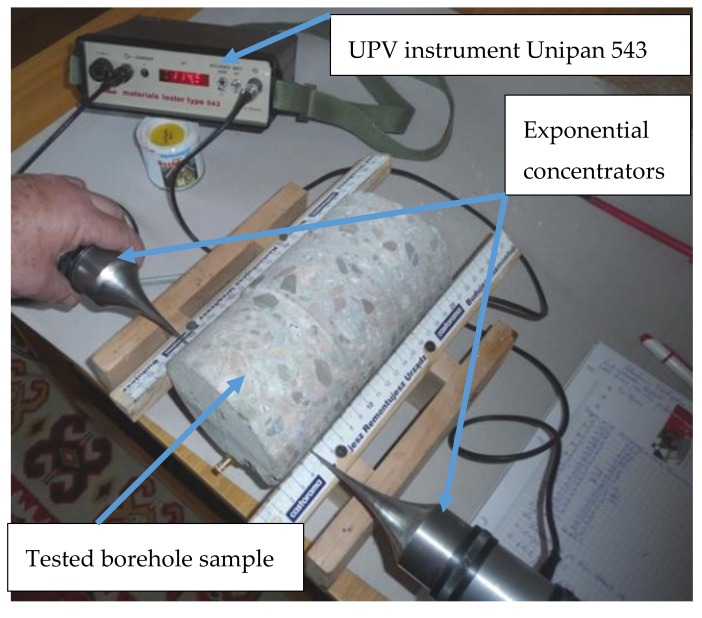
Borehole No. 1 during ultrasound tests.
